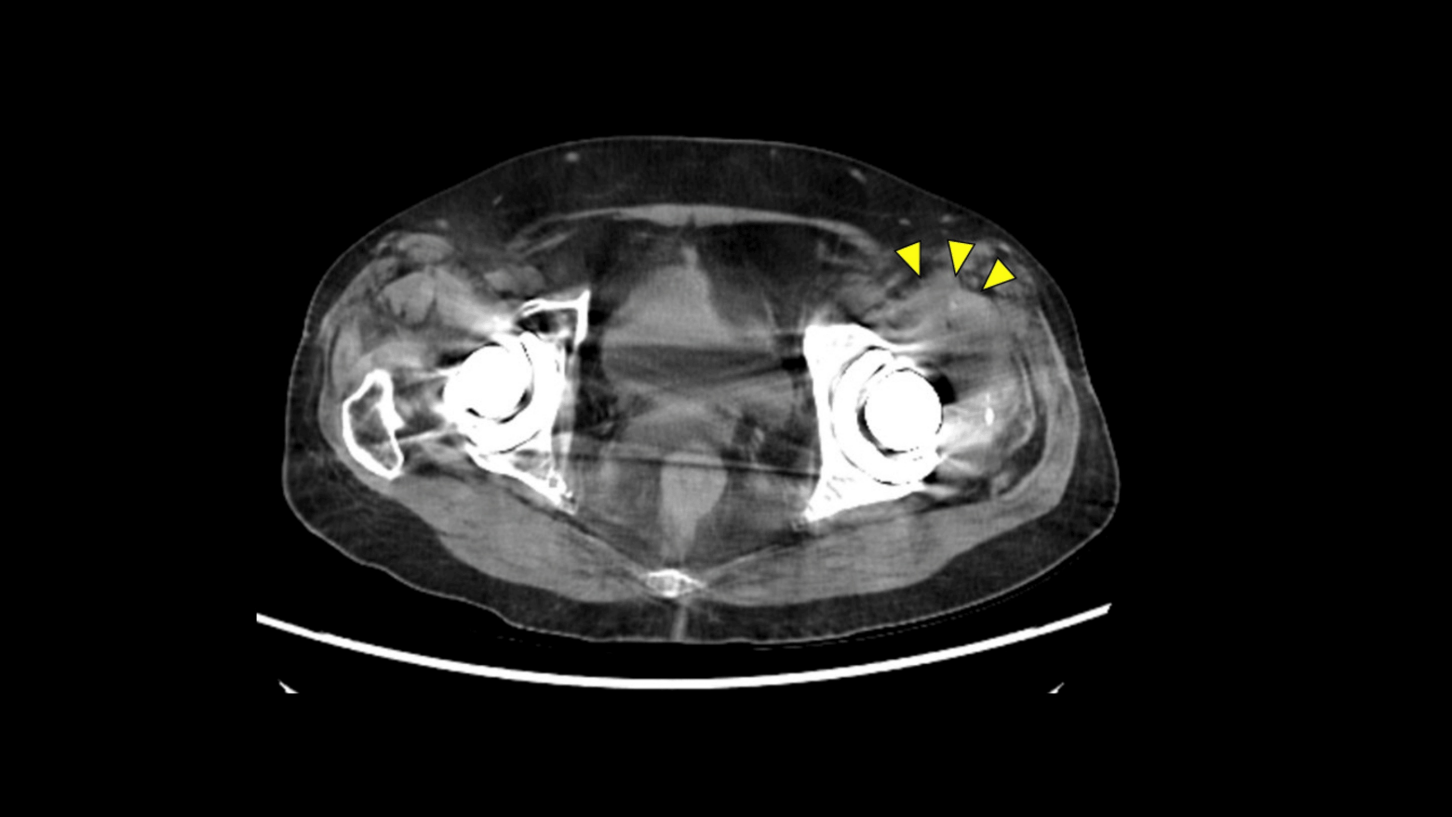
Preoperative computed tomography (CT) scan of the hip Fluid collection is observed in the left hip joint.
